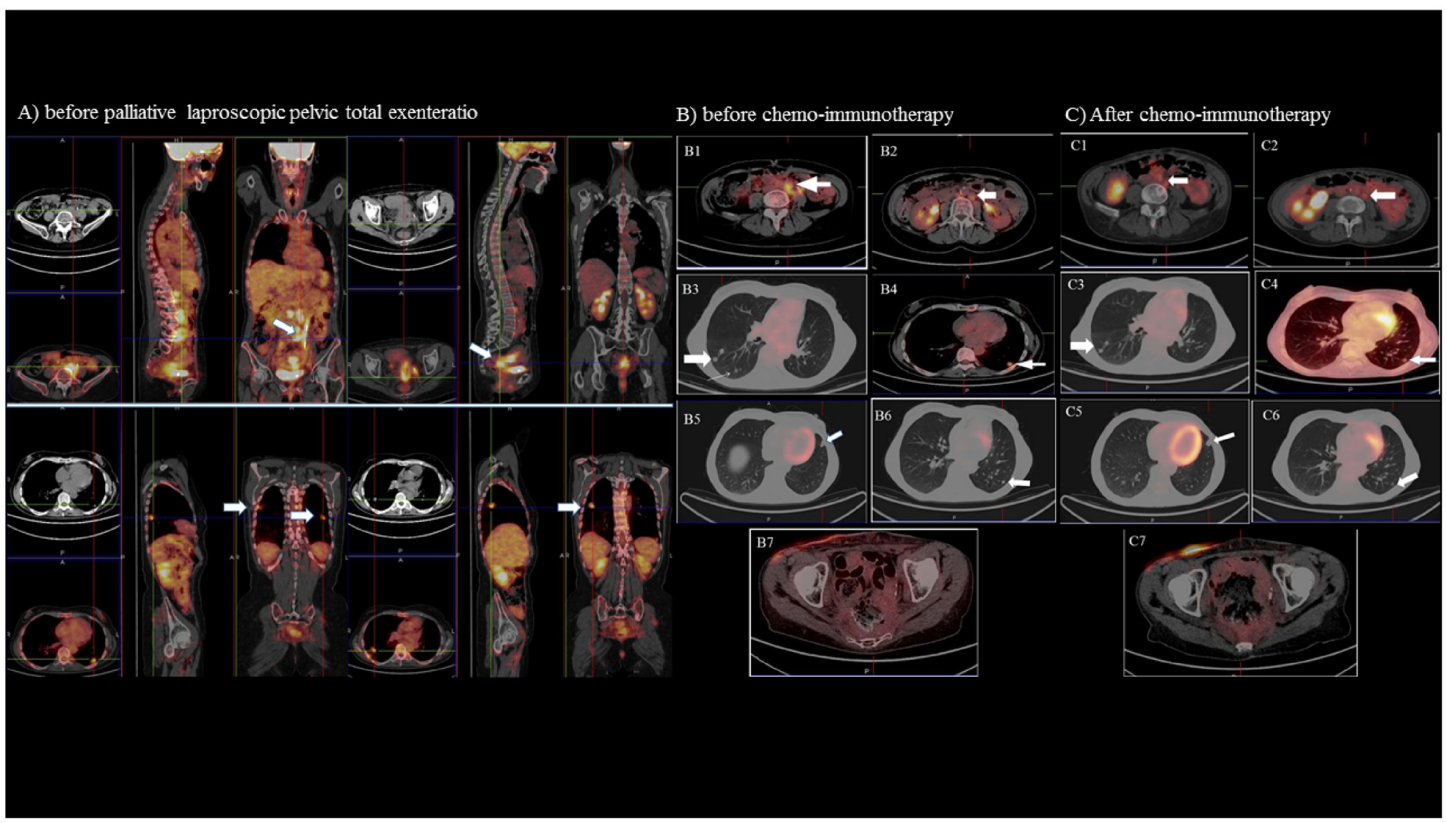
Positron emission tomography (PET) and computed tomography (CT) imaging The imaging of the disease extent before laparoscopic total pelvic exenteratio (Panel A) show in particular in the upper scans a large area of intense hypercaptation of the metabolic tracer in the pelvis and in the lombo-aortic and common iliac lymph node (white arrows); in the lower scans multiple positive areas in the lung (apical segment of the right superior lobe, superior segment of the inferior lobe bilaterally, a medium postero-basal segment of the left inferior lobe (white arrows). (Panel B and C) shows the PET/CT and CT imaging before (panel B) and after (panel C) chemo-immunotherapy of the: 1-2) left lombo-aortic lymphnodes; 3) right lung nodules; 4-6) left lung nodules; 7) pelvic region.
